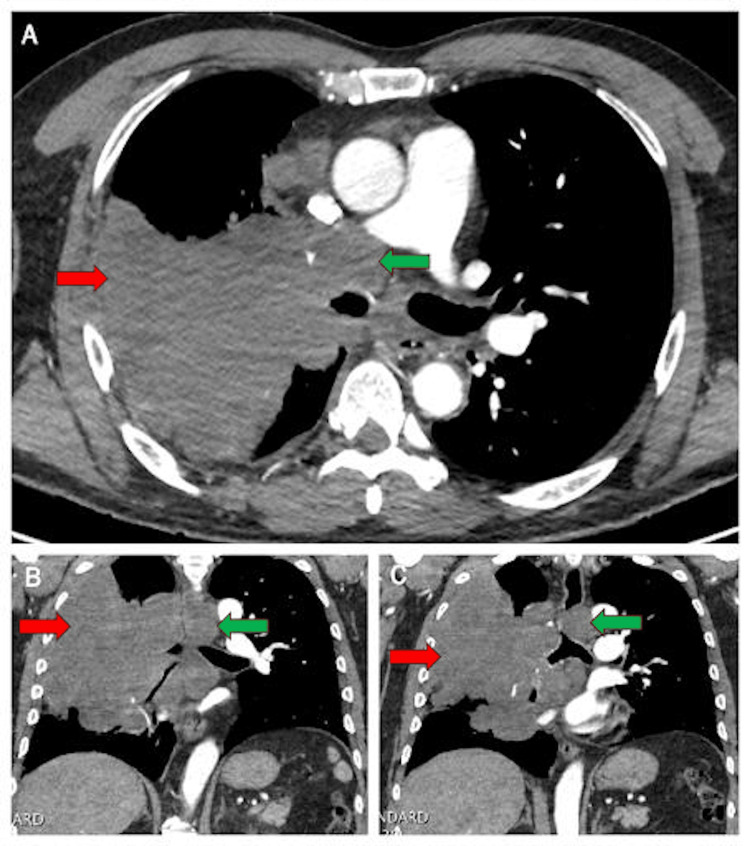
Contrast-enhanced computed tomography (CT) of the chest demonstrating the right hilar and upper lobe mass. Panel A shows an axial view, and panels B and C show coronal views. The mass is indicated with red arrows, and the associated mediastinal lymphadenopathy is highlighted with green arrows. The images demonstrate complete obstruction of the right upper lobe bronchus and extensive lymph node involvement.
